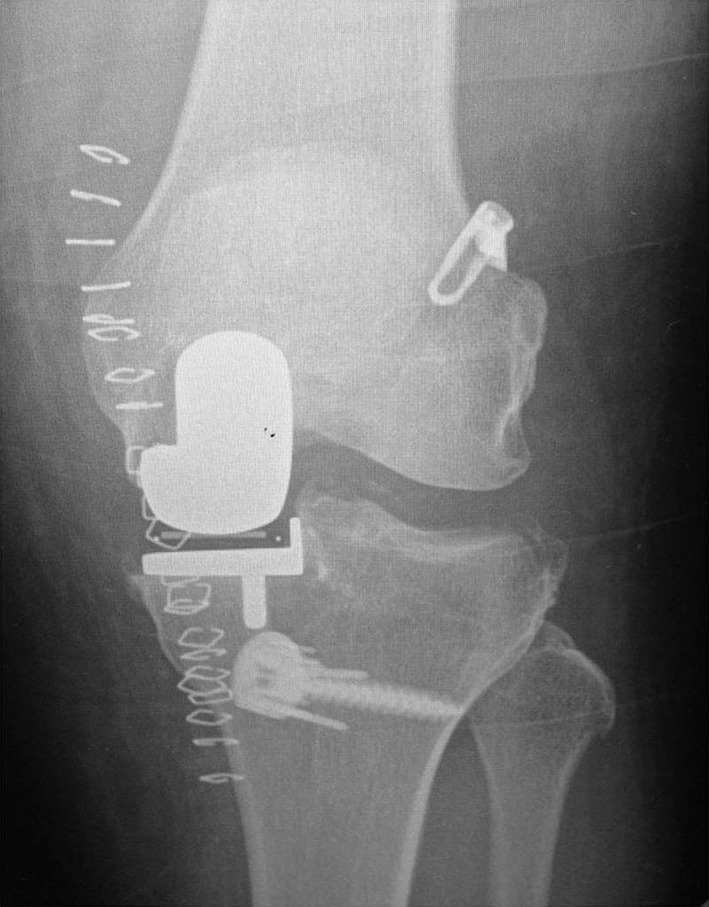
Post-operative X-ray of a combined unicompartmental knee arthroplasty (UKA) and ACL-reconstruction
